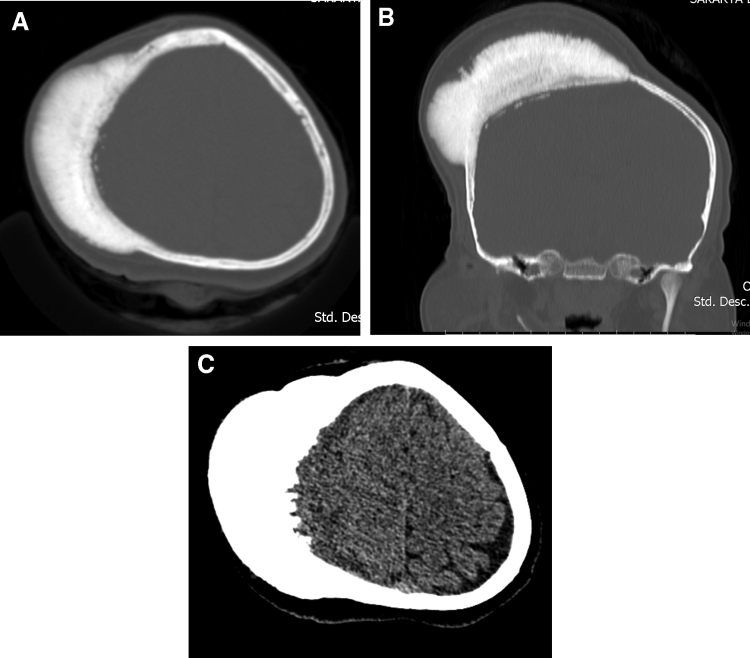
Preoperative cranial computed tomography obtained in 2024. (A) Axial bone-window image showing marked interval enlargement of the right temporoparietal calvarial lesion. (B) Coronal bone-window image demonstrating extensive diploic expansion and cortical thinning. (C) Axial brain-window image showing inner table irregularity abutting the dura without a definite intradural mass component.
